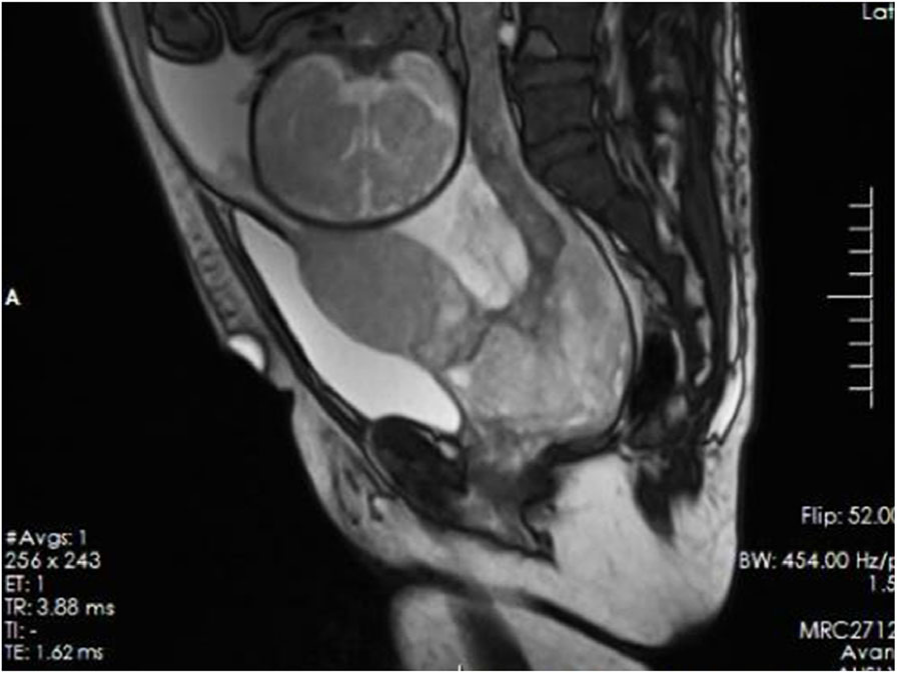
Magnetic resonance imaging (MRI) at gestational week 36^+ 5^ reveals total placenta previa. The placenta is located mainly on the anterior side. A T2 dark band, uterine bulging, myometrial thinning of the anterior wall and myometrium loss between the placenta and bladder wall are observed. Multiple linear processes that had invaded into the myometrium are observed
